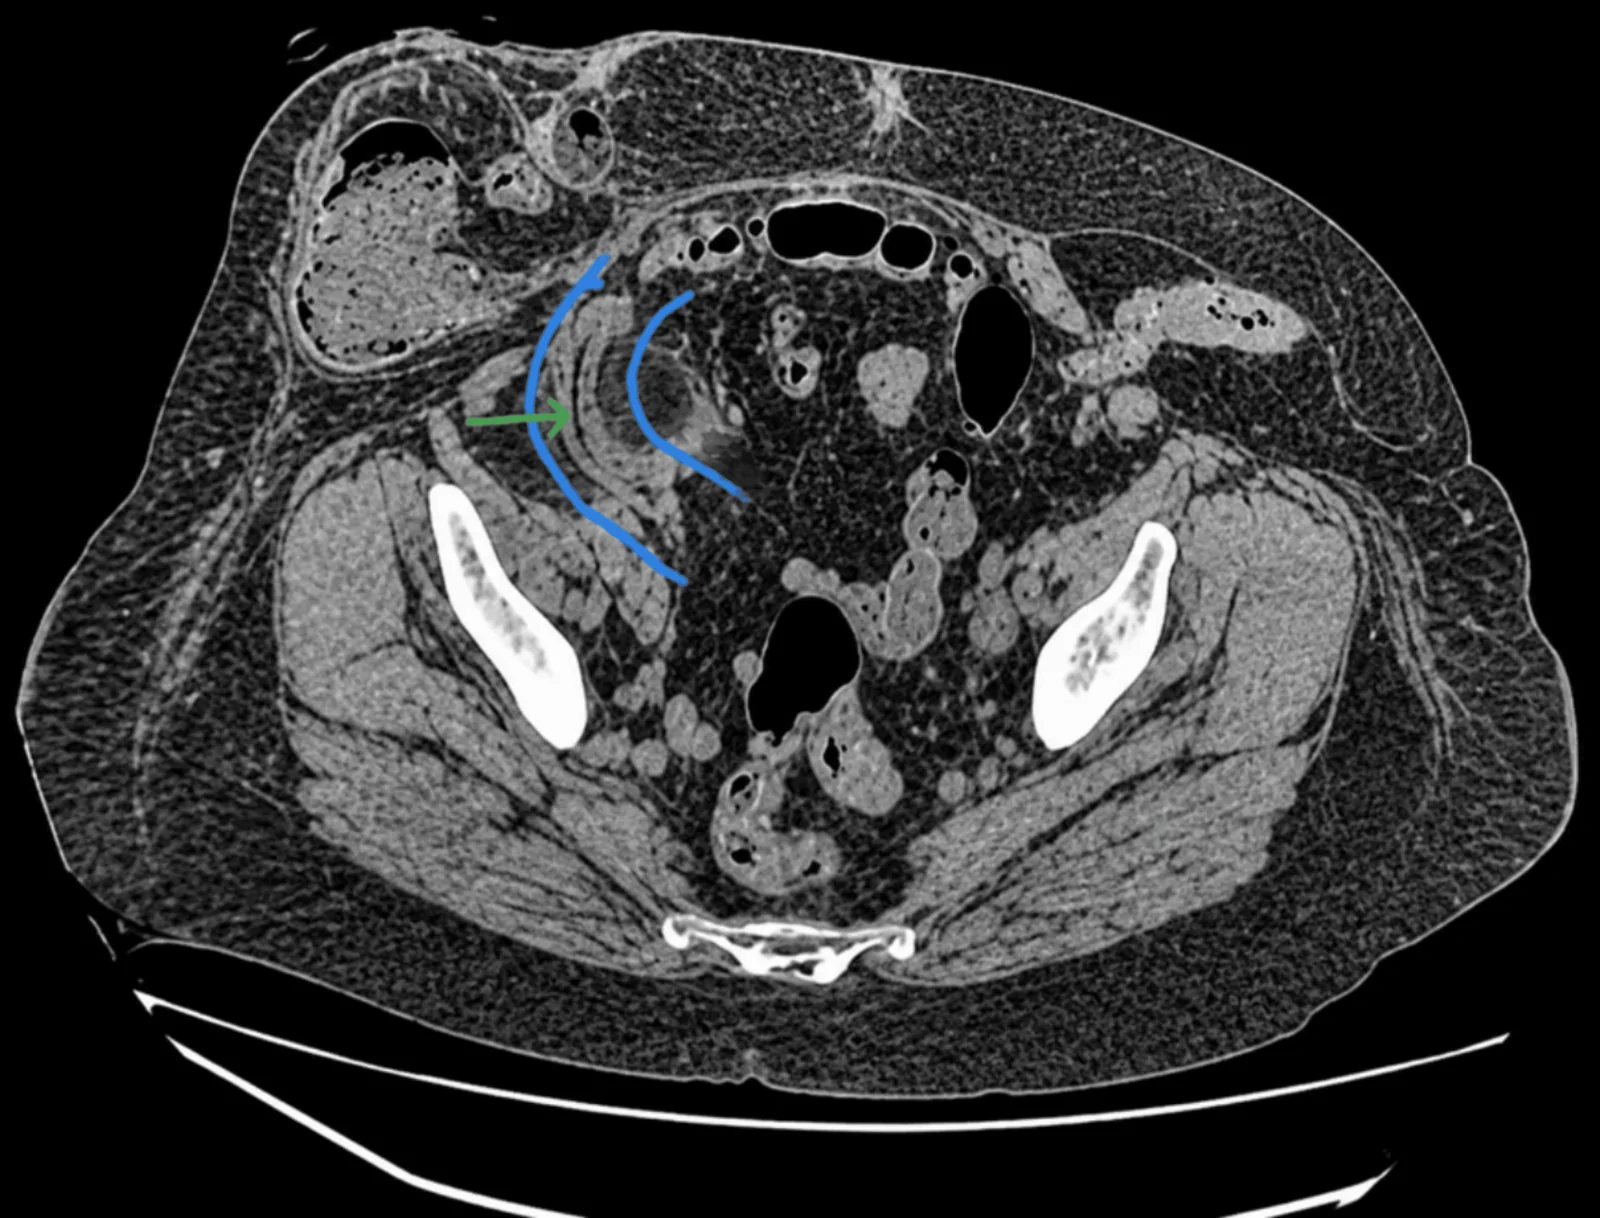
Axial view of the CT abdomen The image shows a collapsed ileal conduit outlined by blue lines, and the green arrow indicates the catheter within the conduit CT: computed tomography
